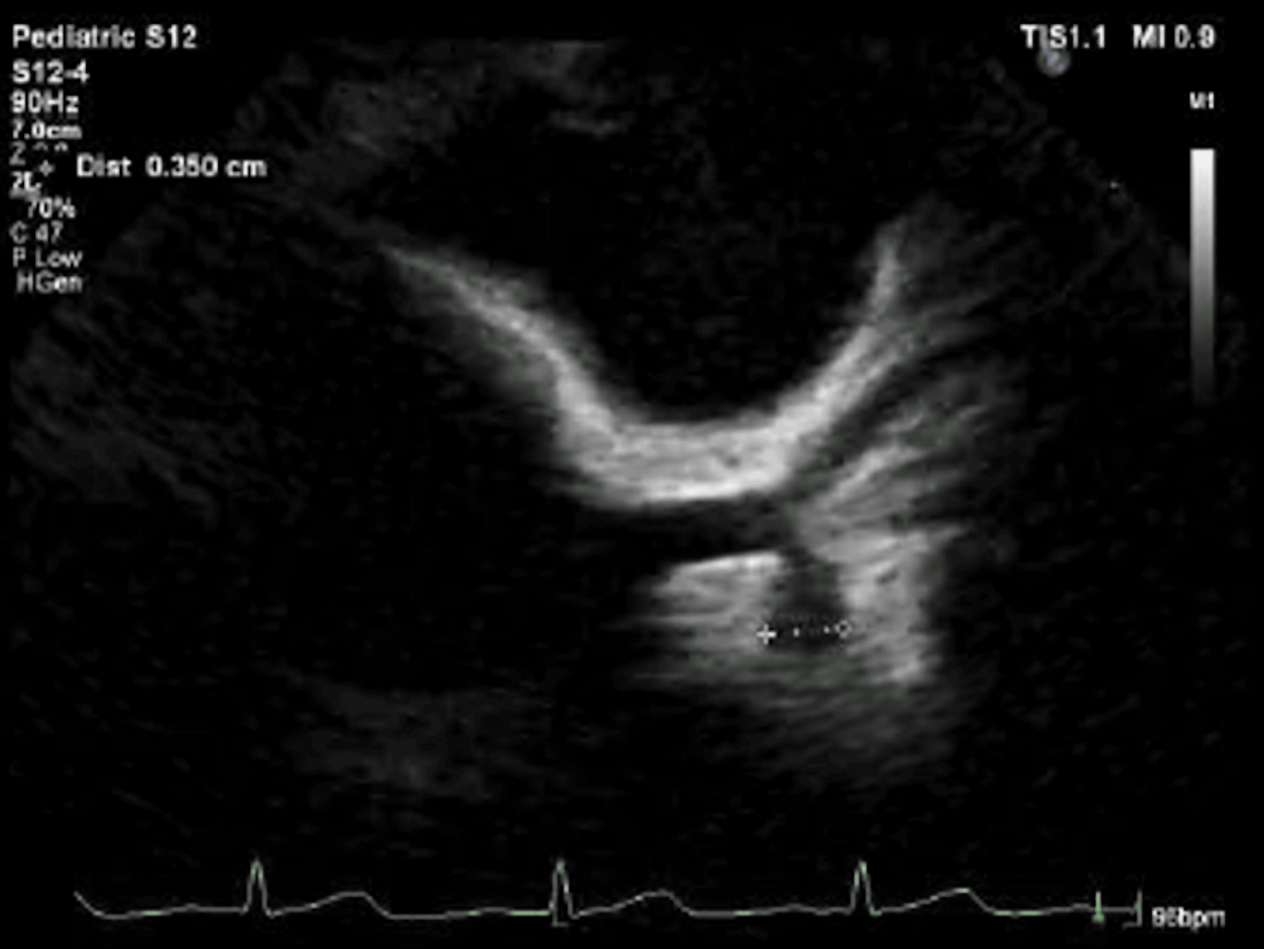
Transthoracic echocardiography on day 10 showing coronary involvement. Left circumflex coronary artery aneurysm (3.5 mm; maximum Z-score 3.95).
